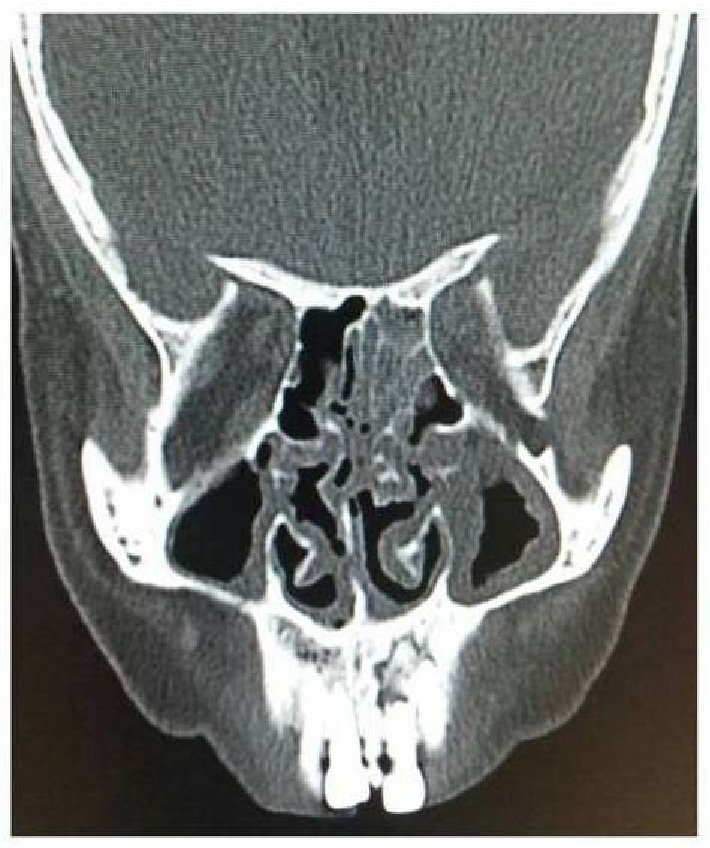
CT scan images shows thickening of mucosa and opacification of the sinuses.
